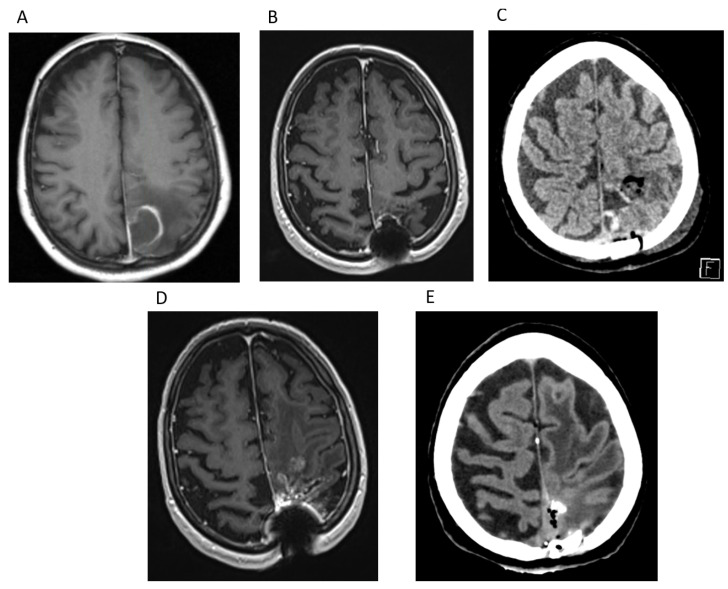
The cranial MRI and CT scan of patient 1. (A) Preoperative T1 contrast-weighted axial image; (B) postoperative routine T1 contrast-weighted MRI axial image, after chemo-, radiotherapy, no signs of recurrence; (C) postoperative routine T1 contrast-weighted MRI axial image. Progression of the lesion with a new cystic component; (D,E) T1 contrast-weighted MRI axial image and CT axial image obtained at the time of admission. Signs of osteomyelitis were present at the previous surgical site, including bone erosion and air pockets.
